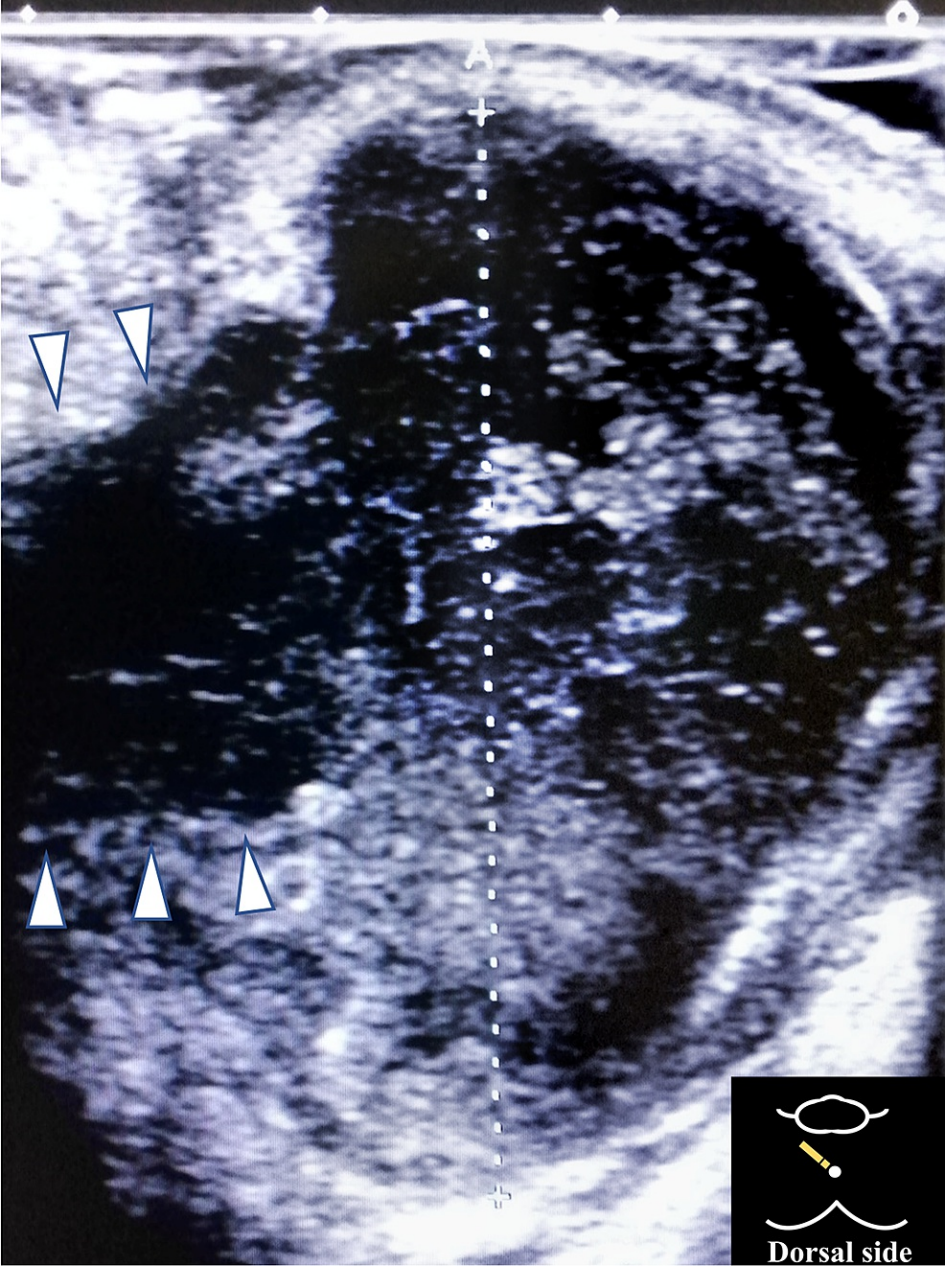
Ultrasound findings The ultrasound probe (linear probe 7.5 Hz) was placed perpendicular to the tender and swollen skin around the anus. It revealed a heteroechoic subcutaneous mass with a hyperechoic rim (diameter 38 mm). Fistulas were observed as hypoechoic tracts (arrowhead) that were continuous with the abscess.
